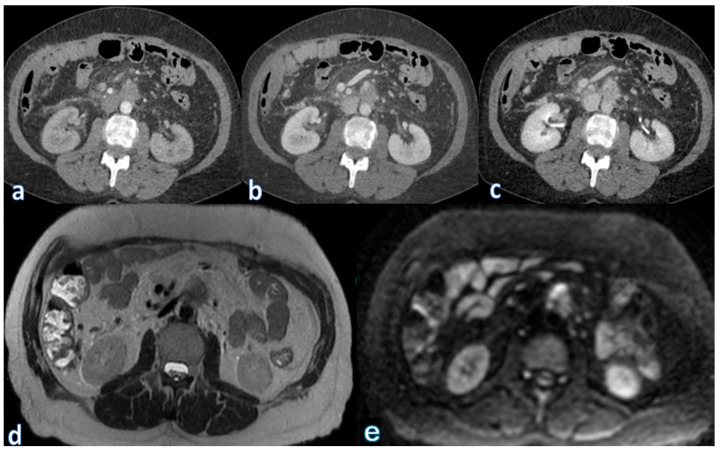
Axial CT images in the arterial (a), venous (b), and late (c) phases and T2-weighted MR (d) and DWI (e) images showing inhomogeneous solid tissue in the periaortic area as if resulting from local recurrence. This finding was observed in a patient who underwent major pancreatic resection for adenocarcinoma of the head of the pancreas three months after surgery.
